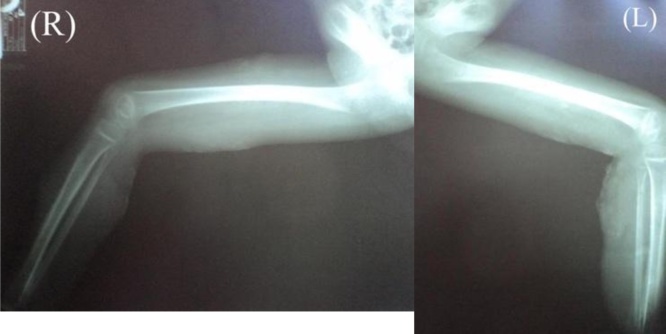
X-Ray of the Patient’s Lower Extremities showing no signs of bone and surrounding inner soft tissue destruction.
